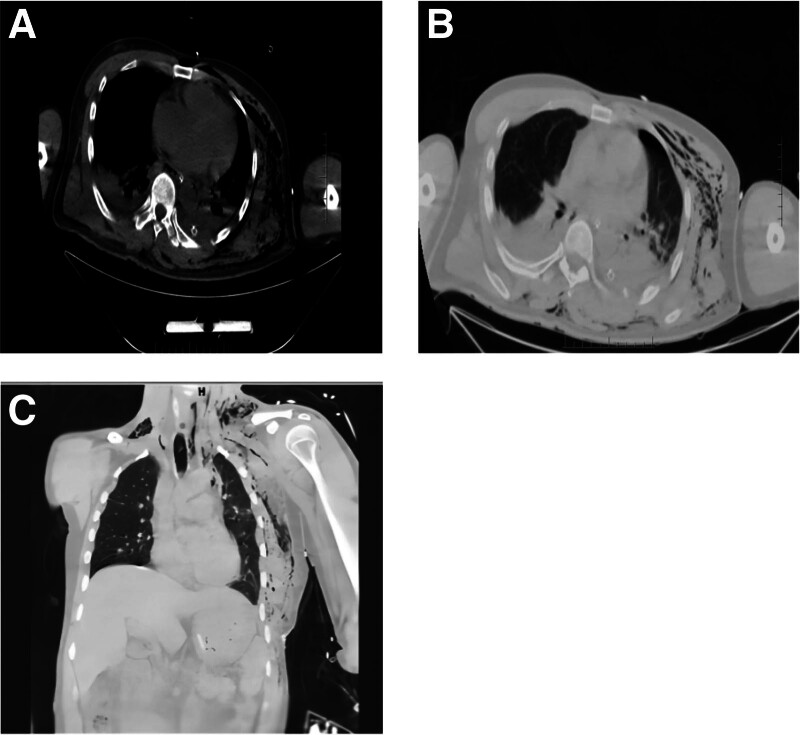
Postoperative chest and abdominal CT. (A, B) Small bilateral pleural effusion, left lower lung consolidation. (C) Postoperative stomach and spleen repositioned in the abdominal cavity, mediastinal and cardiac repositioning. CT = computed tomography.
